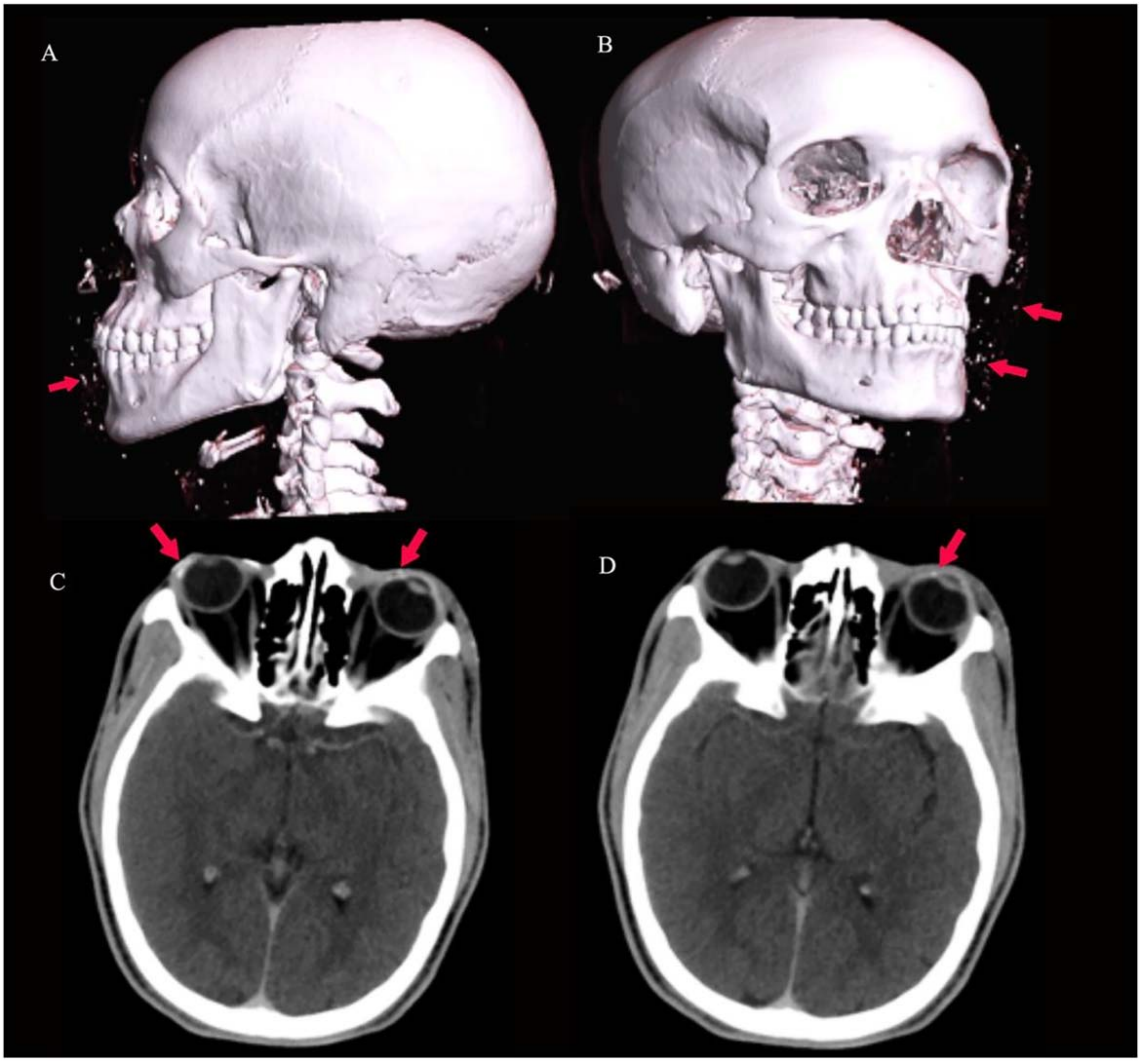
3D virtual reconstruction images (A and B) show multiple radio-opaque foreign bodies in the subcutaneous tissue of the face, as shown by arrows. Axial CT of the brain (C and D) at the level of the orbits shows conjunctival foreign bodies (shrapnel), as shown by arrows.
